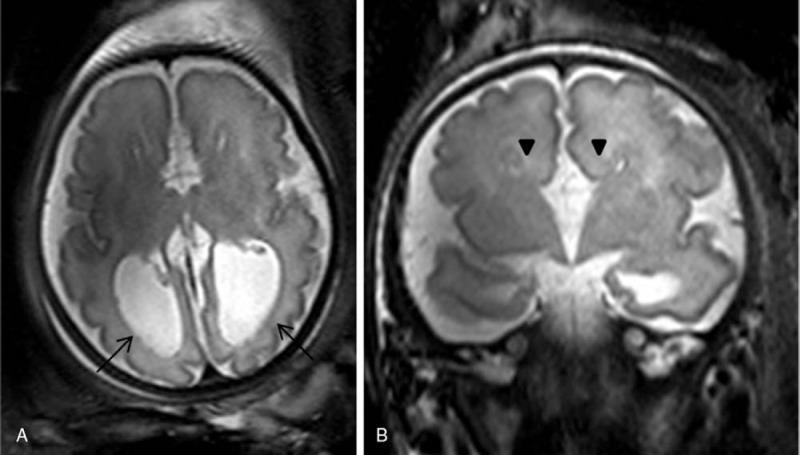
Agenesis of the corpus callosum in a fetus at 35 weeks gestation. Axial and coronal T2 turbo-spin echo (TSE) single-shot magnetic resonance imaging (MRI) scans show absence of the corpus callosum and colpocephaly (arrows, A). Probst bands (arrowheads, B).
